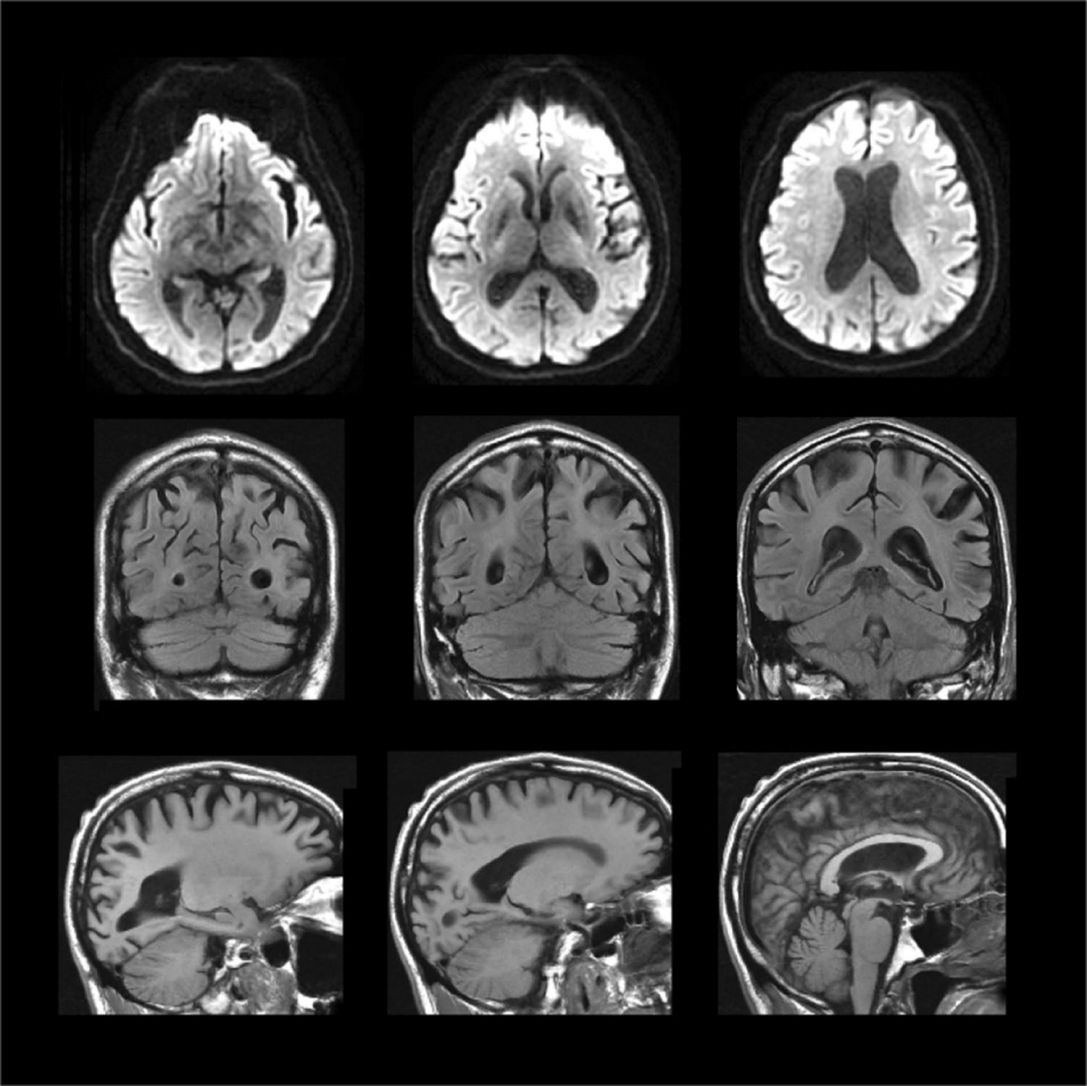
Clinical T1 weighted MRI image of AB’s brain acquired on a 1.5 T scanner showing structural abnormalities and the thinning of the cortex in the posterior of the brain. Ventricular enlargement was also observed
